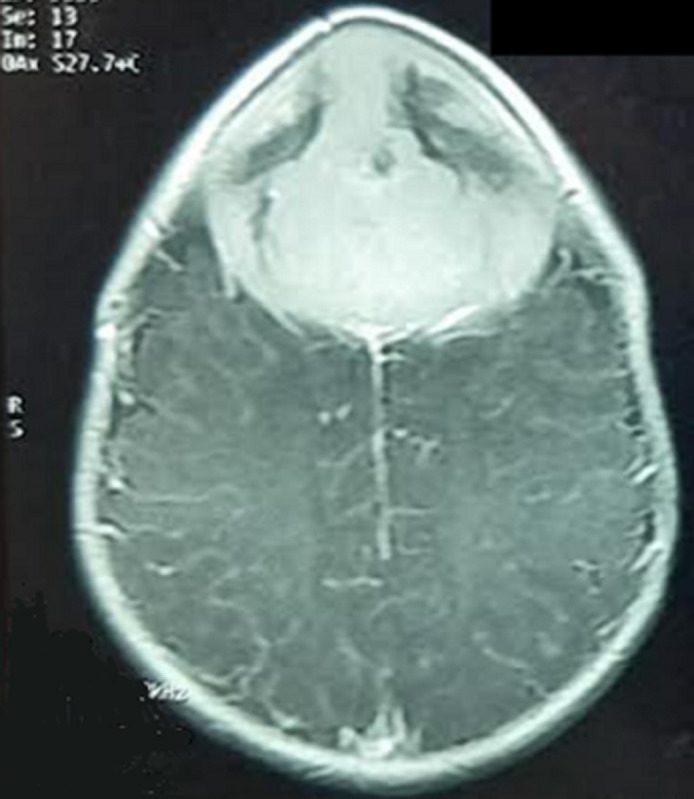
cerebral magnetic resonance imaging axial plane in T1-weighted sequence with contrast
